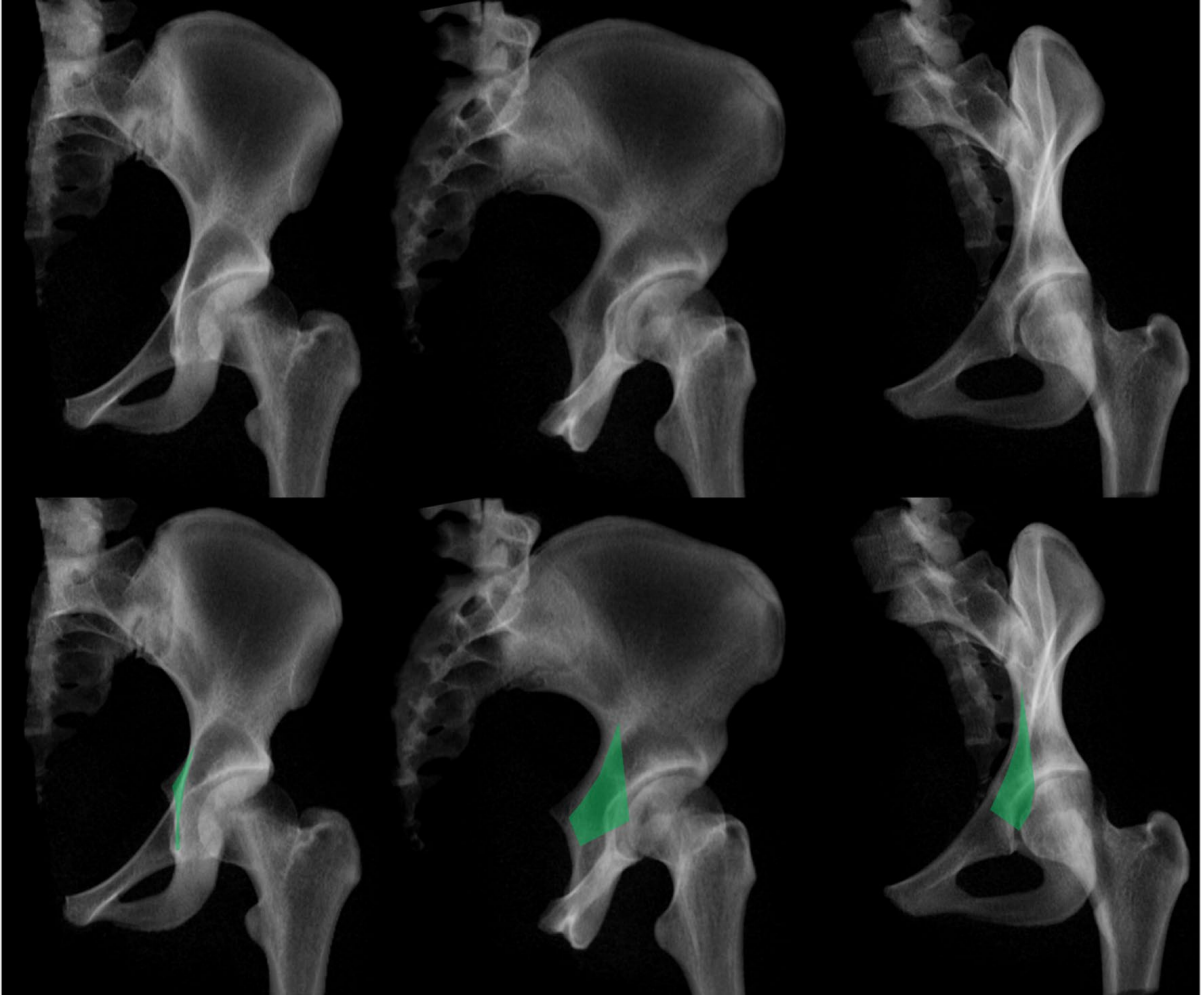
True radiographic appearance of the. QLP on standard acetabular views (AP view, iliac oblique and obturator oblique view)
